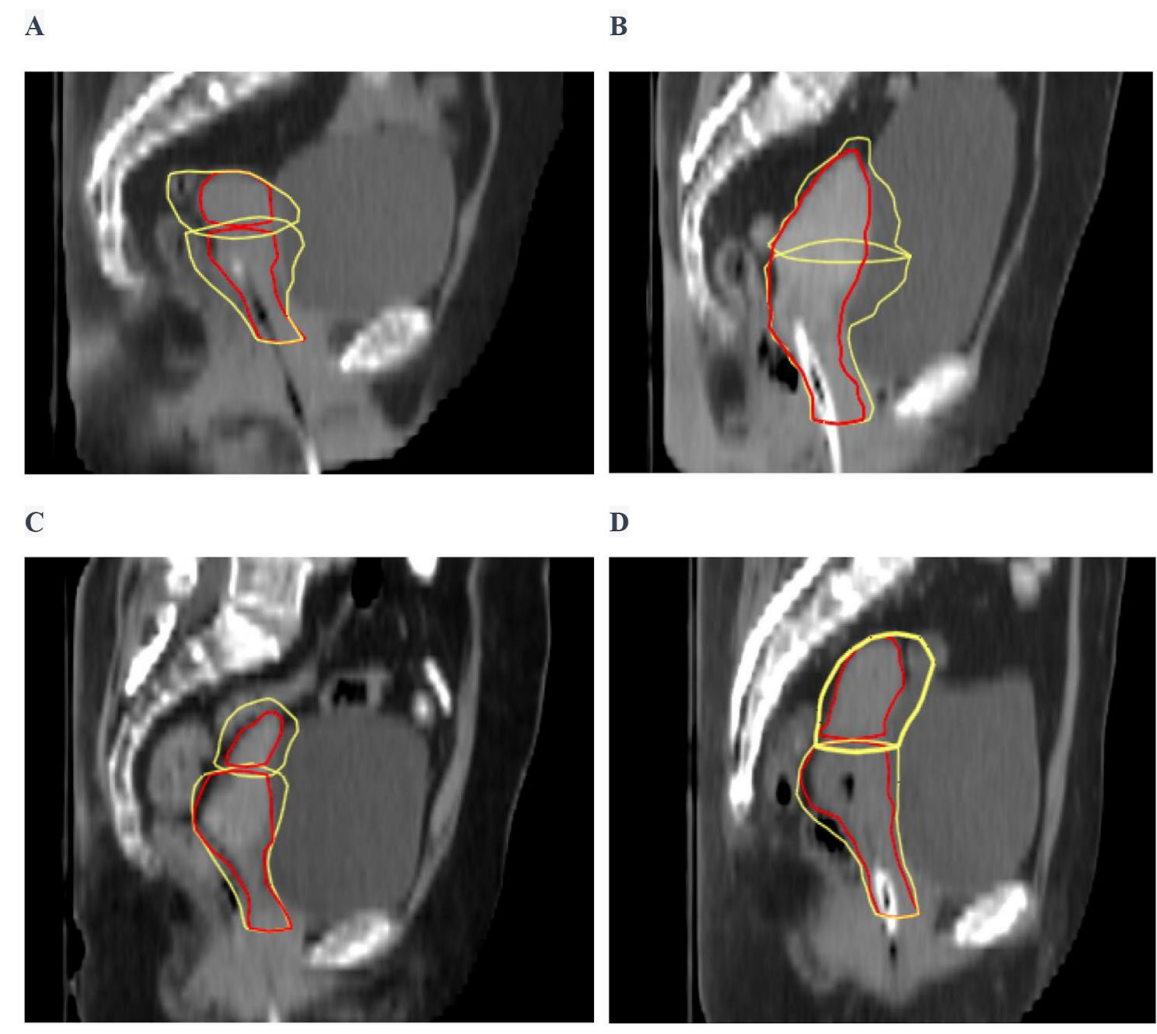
(A) Sagittal image of a planning CT scan from cervical cancer with stage T1. (B) Sagittal image of a planning CT scan from cervical cancer with stage T2. (C) Sagittal image of a planning CT scan from cervical cancer with stage T3. (D) Sagittal image of a planning CT scan from cervical cancer with stage T4. Initial CTV-U and CTV-C are shown in red, ultimate CTV-U and CTV-C are shown in yellow
